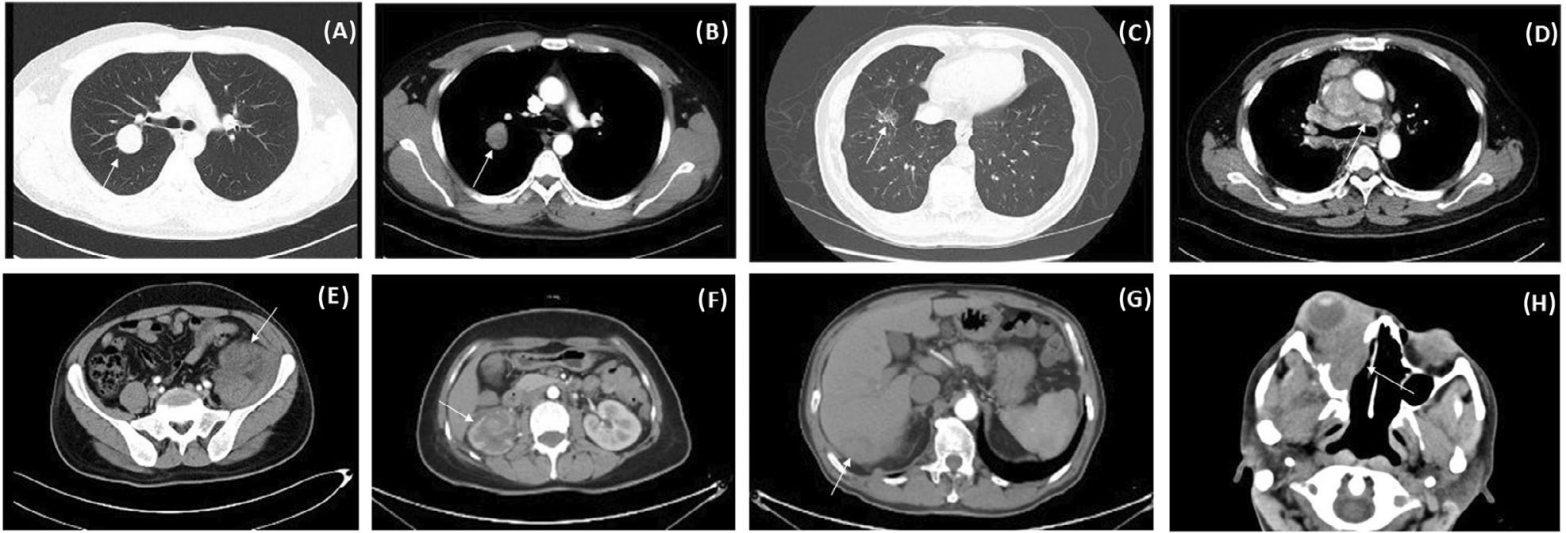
The CT scans of an inflammatory myofibroblastic tumor. (A and B) The CT scan showed a well‐delineated nodule in a patient with a pulmonary inflammatory myofibroblastic tumor. (C) The CT scan showed a ground glass nodule in a patient with a pulmonary inflammatory myofibroblastic tumor. (D) The CT scan showed hilar and mediastinal lymph nodes in a patient with a pulmonary inflammatory myofibroblastic tumor. (E) The contrast‐enhanced CT scan demonstrated heterogeneous enhancement of the left abdominal inflammatory myofibroblastic tumor. (F) The contrast‐enhanced CT scan showed homogeneous enhancement of the right renal inflammatory myofibroblastic tumor. (G) The contrast‐enhanced CT scan demonstrated heterogeneous enhancement right hepatic inflammatory myofibroblastic tumor. (H) The contrast‐enhanced CT scan showed a right nasal sinus inflammatory myofibroblastic tumor involving the orbit.
